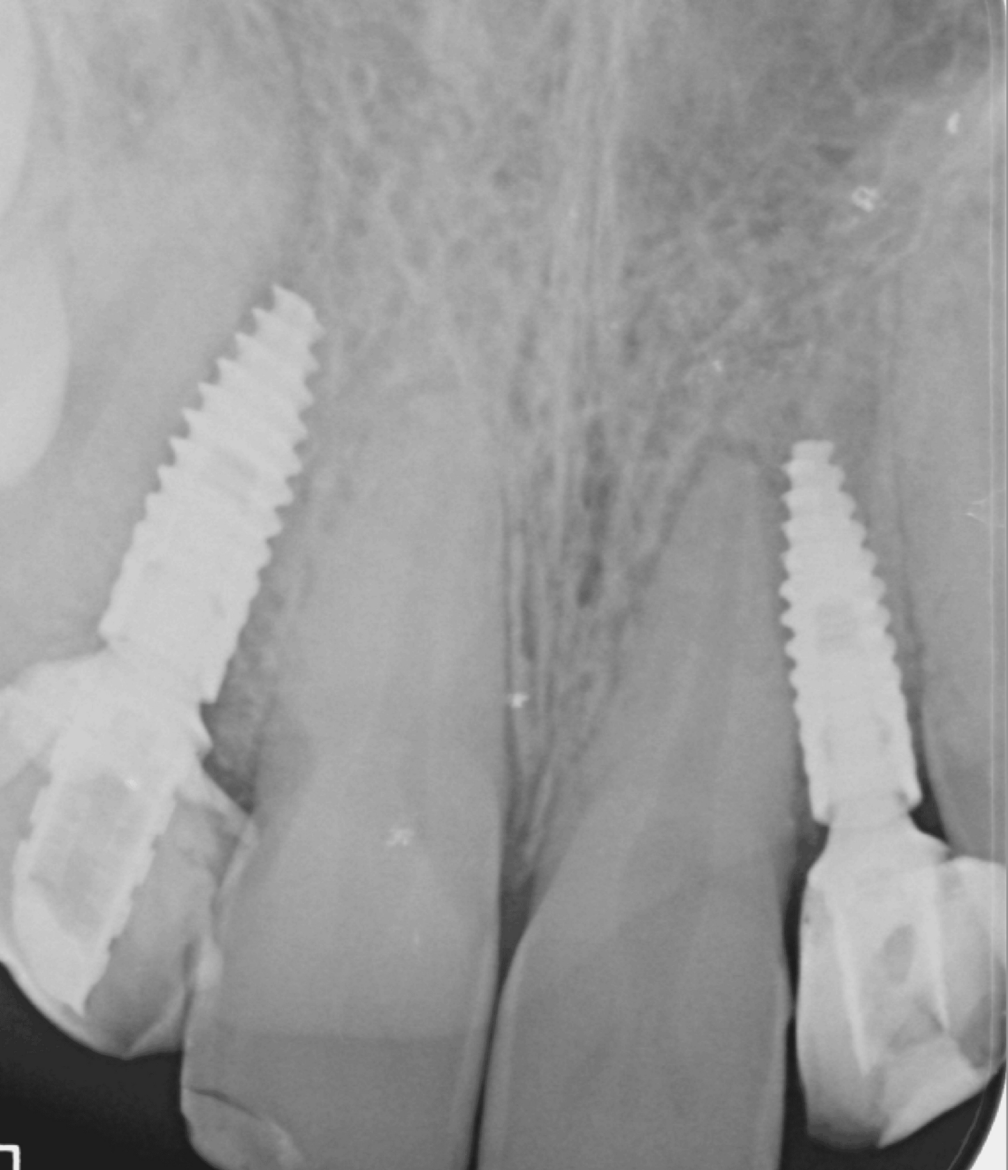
X-ray confirming the seating of the implant and the provisional crown
